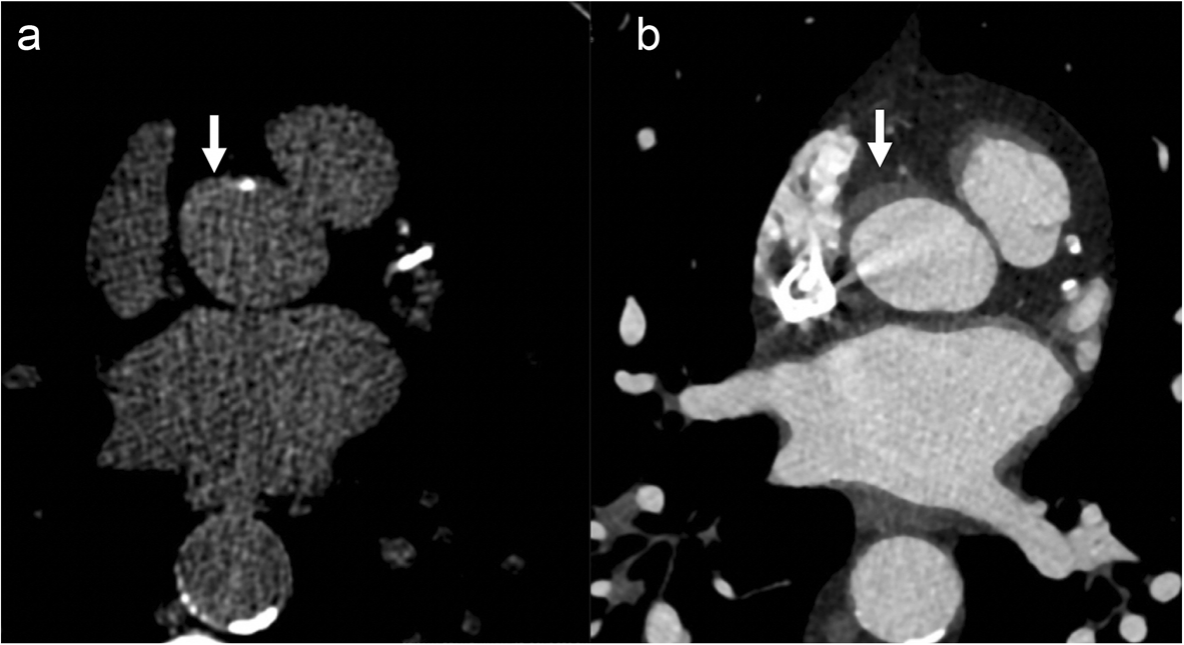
A 72-year-old male with chest pain after conventional angiography done as a part of valve-in-valve surgical clearance. Non-contrast (a) and contrast-enhanced (b) CTA images showing hyperdense aortic wall thickening at the sinotubular suggesting focal intramural hematoma, related to intimal injury from difficult right coronary artery cannulation
